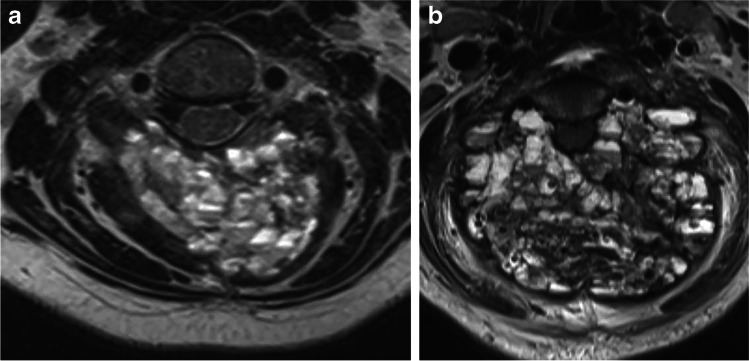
Axial MR images in a 10-year-old girl (patient 13) with an aneurysmal bone cyst (ABC) of the posterior half of the C3 ring. a Axial MR image 2 weeks before the first treatment shows expansion and replacement of spinous process and bilateral lamina at C3. More aggressive ABCs tend to have innumerable tiny cysts, as depicted in this case. b Axial MR image at the same level 3 months later shows near doubling in size of this very aggressive lesion, now with effacement of the canal
